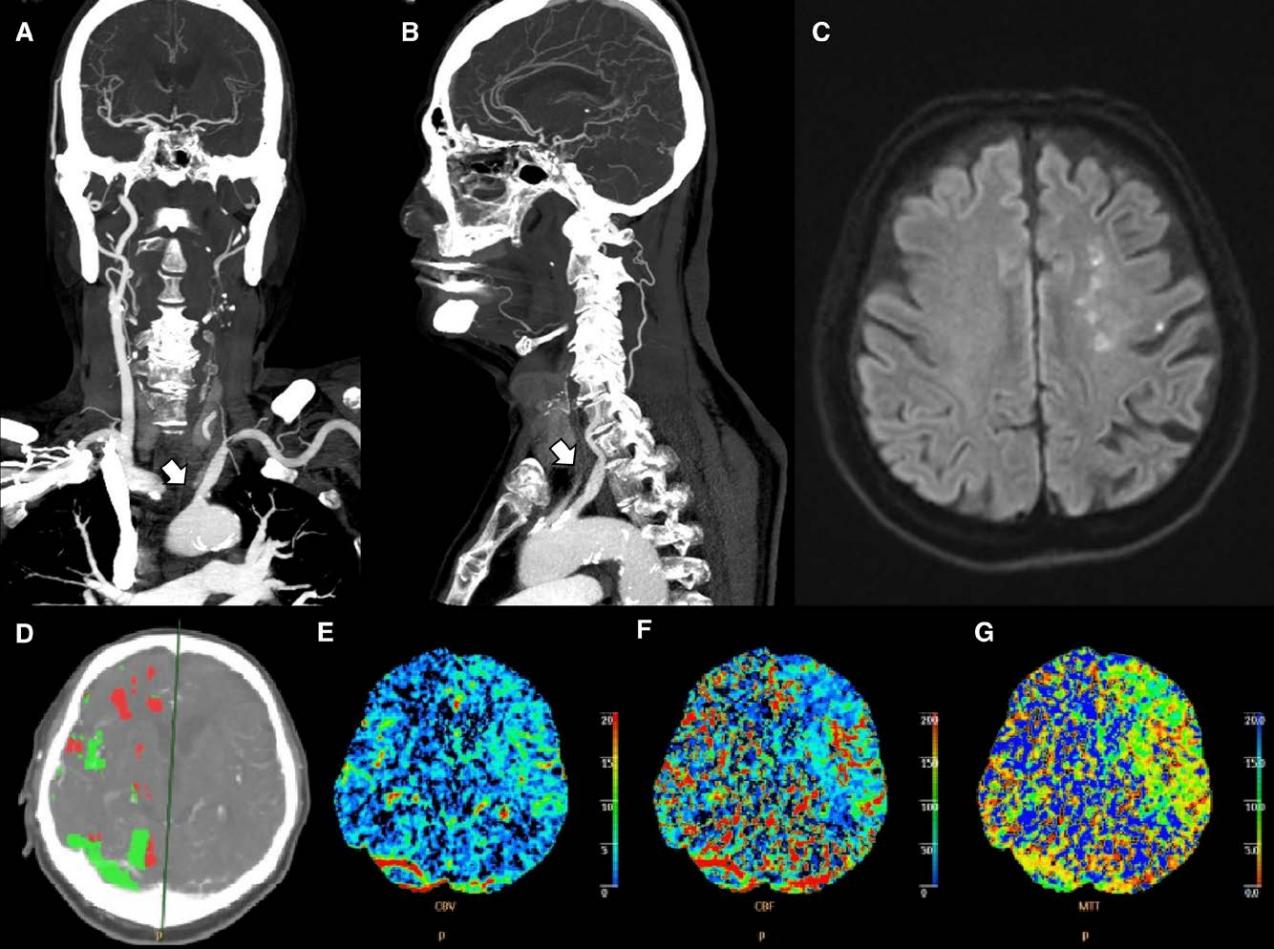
Left middle cerebral artery territory infarction with left CCA occlusion (Case 2). (A) Left common carotid artery (CCA) occlusion (arrow) in coronal computed tomography angiography (CTA) imaging. (B) Left CCA occlusion (arrow) in sagittal CTA imaging. (C) The diffusion restriction of the left internal carotid artery (ICA)-middle cerebral artery (MCA) internal border zone in (D–G) diffusion-weighted magnetic resonance imaging. Perfusion CTA images show increased mean transit time and decreased cerebral blood flow and blood volume on the right hemisphere. CCA = common carotid artery.
